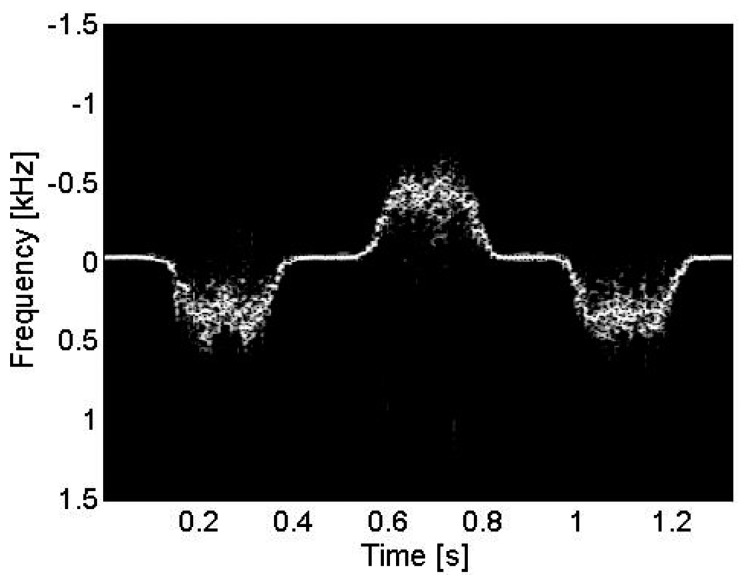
PW Doppler imaging of a moving reflector.
